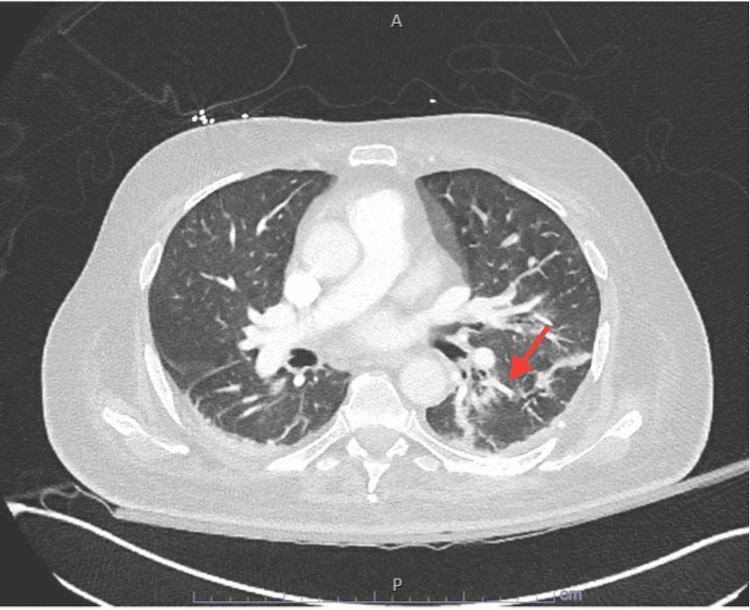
CTA of chest showing moderate streaky and consolidative opacities of bilateral lower lobes, with a minimal bilateral pleural effusion. No pulmonary embolus is seen. Red arrow shows streaky opacities seen in the left lung CTA: computed tomography angiogram
